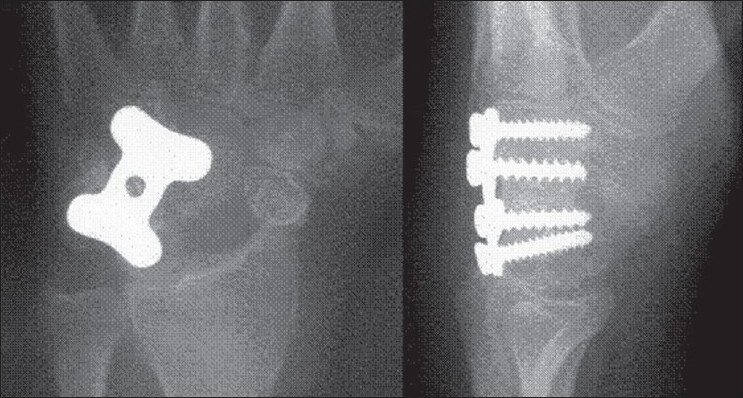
Antero-posterior and lateral radiograph showing four-corner fusion, with H-plate in place
